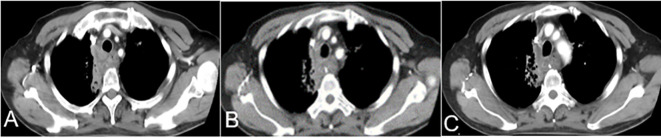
One month after TAIC, (A–C) CT showed a soft tissue density shadow on the right side of the trachea at the thoracic entrance, protruding into the trachea. Middle tracheal stenosis had improved. CT, computed tomography; TAIC, transarterial infusion chemotherapy.
